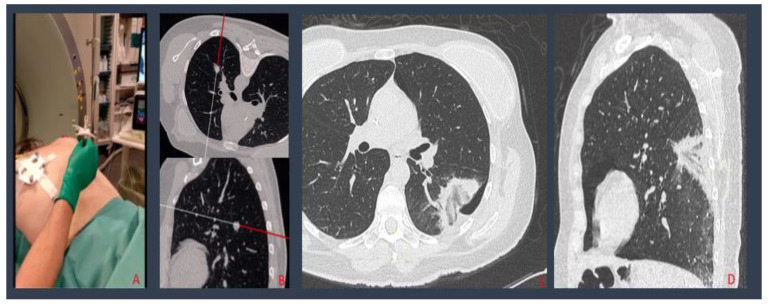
Percutaneous lung MW ablation of a breast cancer metastasis: needle and patient tools positioning (A); the navigation system, creating a 3D virtual model of the patient’s chest, where needle progressions into the patient’s chest are shown (B); post procedural MPR CT reconstructions displaying a small PNX adjacent to the ablation zone, without evidence of residual disease (C,D).
